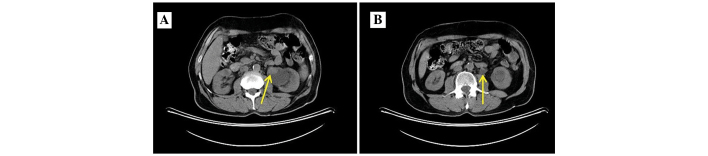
Computed tomography imaging. The axial reconstruction shows (arrow) the recurrence of upper urinary tract urothelial carcinoma in the (A) pelvis renalis and (B) proximal ureter.
